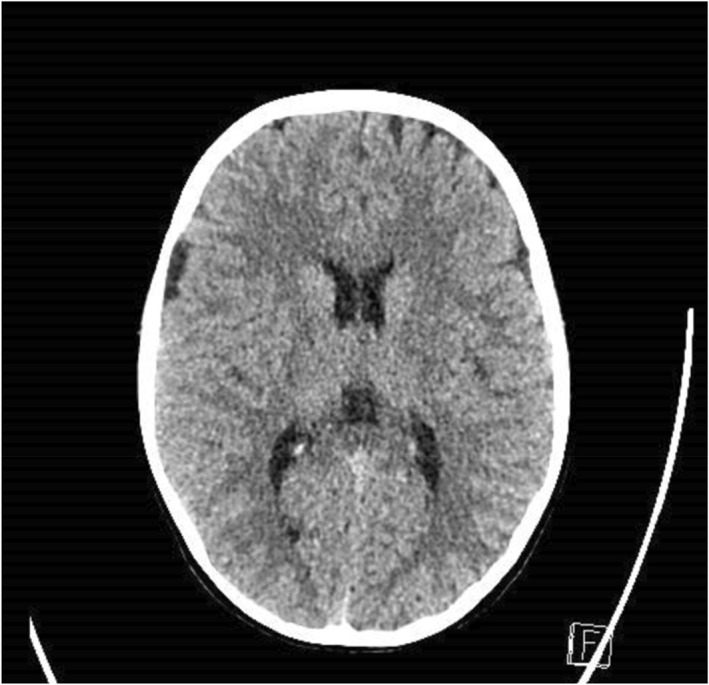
CT brain scan did not reveal any intracranial lesions or bleeds. CT, computed tomography.
